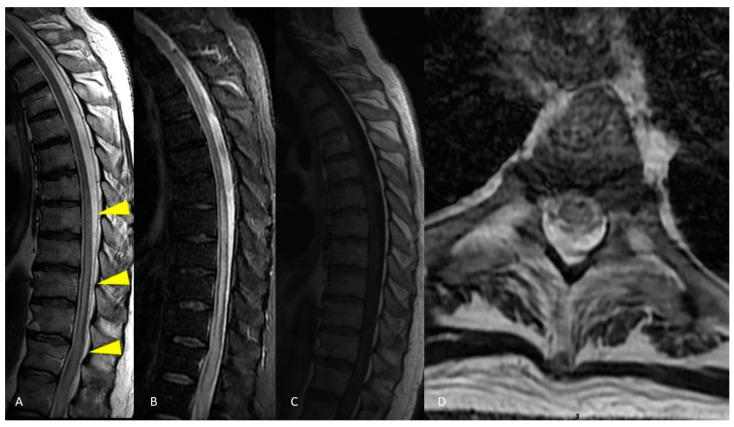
MRI of the thoracic spinal cord. Sagittal T2-weighted images demonstrate long segment hyperintensity of the thoracic cord without expansion or enhancement (A–C). Axial T2-weighted image shows large hyperintensity affecting all the cross-sectional area of the spinal cord (D).
